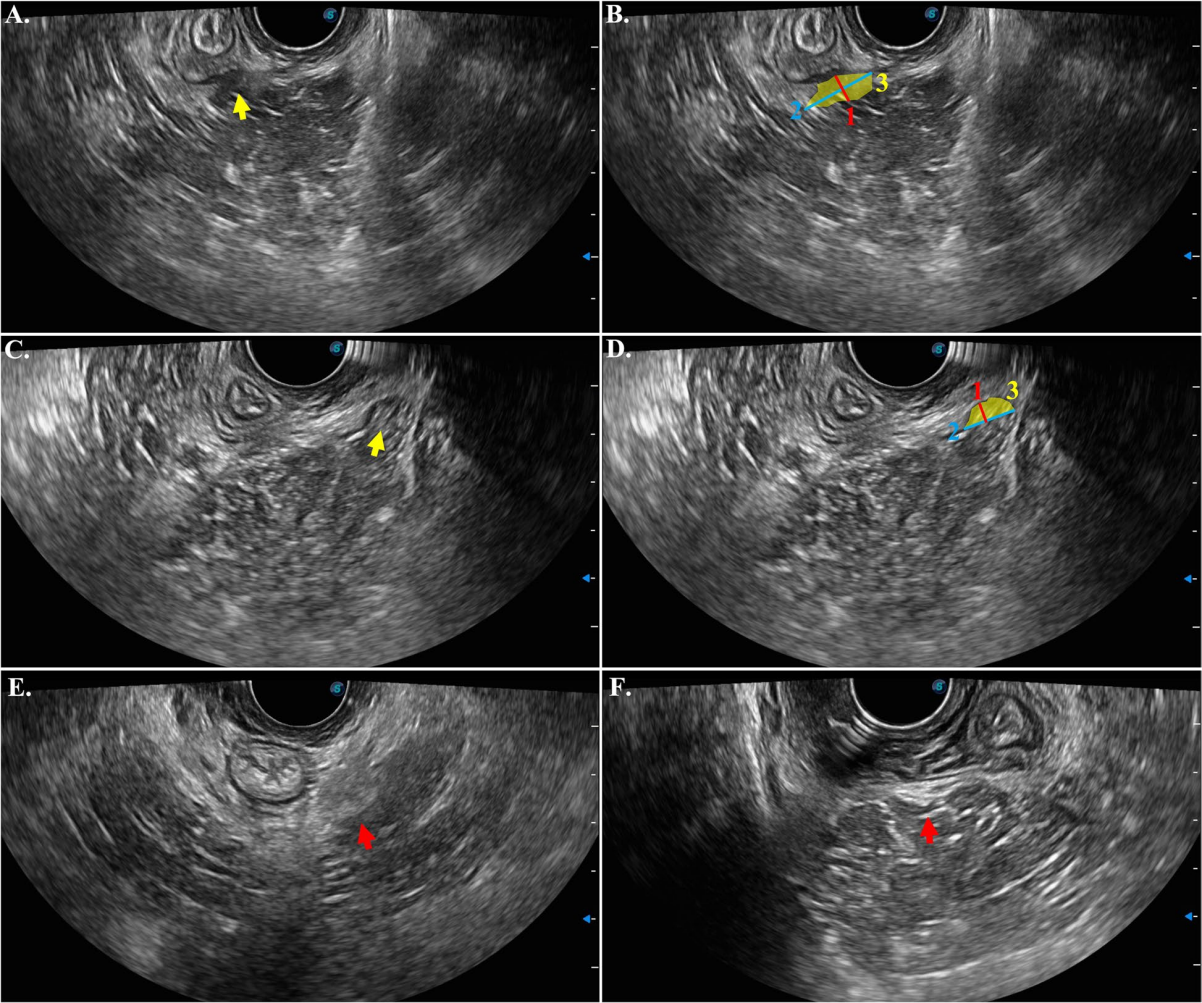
Deficiency (A-D) and focal thickening (E, F) of the puborectalis (PR) in transvaginal ultrasound. Images were from 4 different women with PFMP, and their symptoms of them were left PR pain (A, E), right PR pain (F), and left iliococcygeus pain (C). Yellow arrows (A, C) indicate deficiency. B, D. Measurements of deficiency: 1. width of deficiency; 2. length of deficiency; 3. area of deficiency, indicated by yellow shade. Red arrows (E, F) indicate focal thickening
